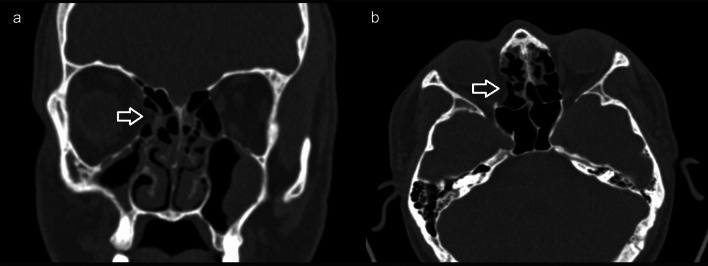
52-Year-old female patient with lamina papyracea dehiscence on the right (arrow) on coronal (a) and axial (b) paranasal CT image
